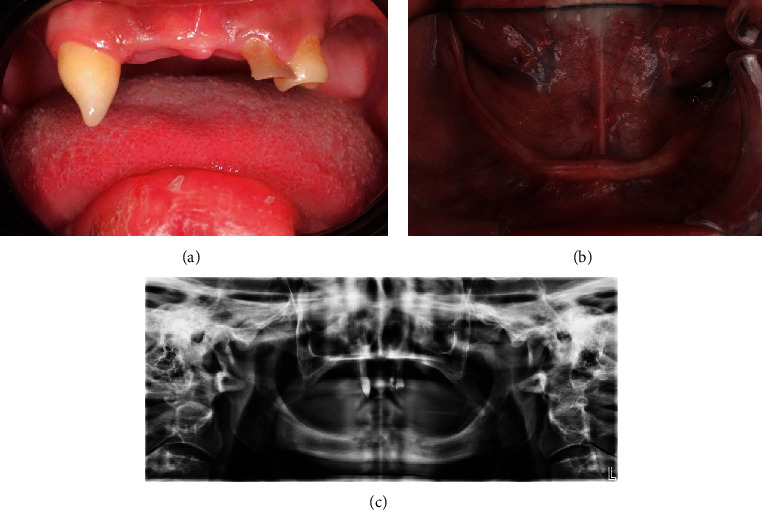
(a) Initial presentation maxillary arch. (b) Initial presentation mandibular arch. (c) Panoramic radiograph.
